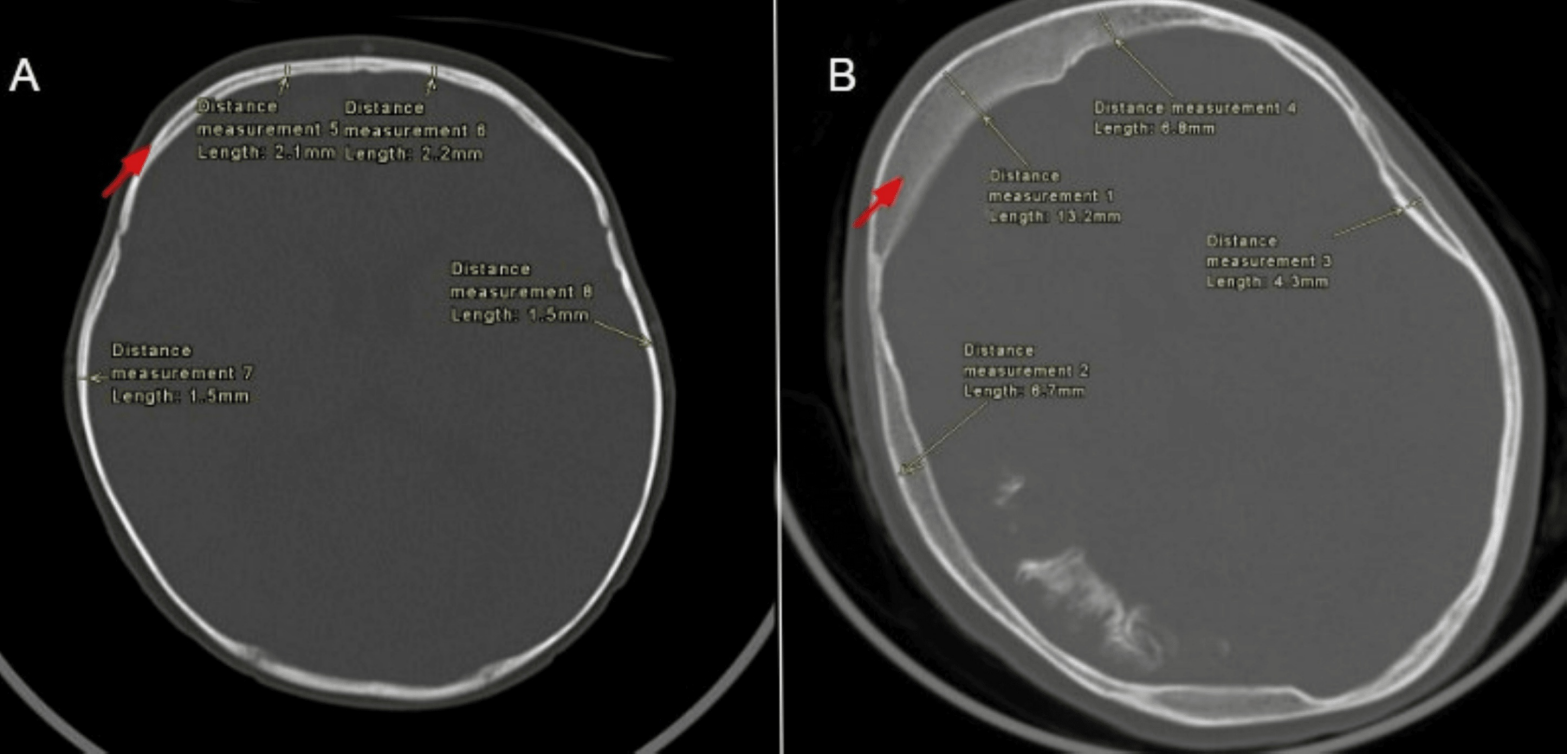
Axial CT bone window images at one year and 11 years of age Axial CT bone window images at one year (A) and 11 years (B) demonstrate progressive right-sided bony hemi-calvarial hypertrophy (red arrows), confirmed by interval increase in calvarial thickness measurements, i.e., from the initial 1-2 mm to follow-up thickness of 4-14 mm.
